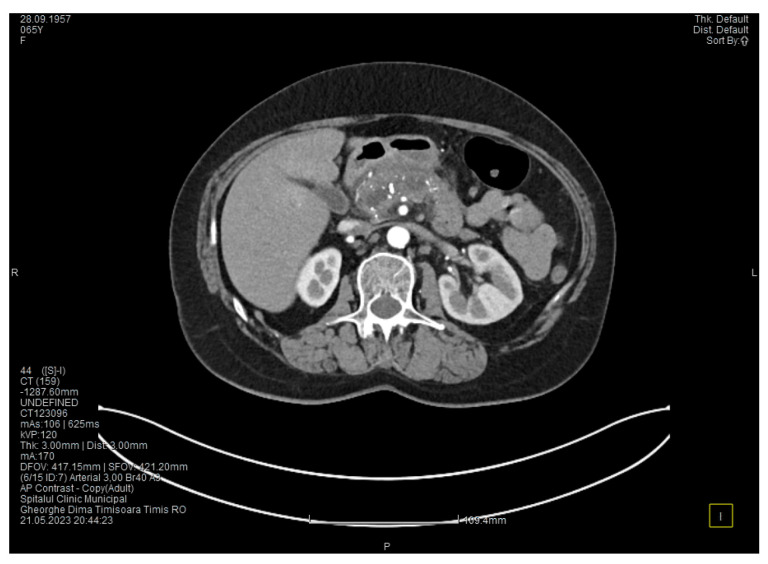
The second CT evaluation shows a dilated Wirsung duct and calcifications in the pancreatic tissue.
